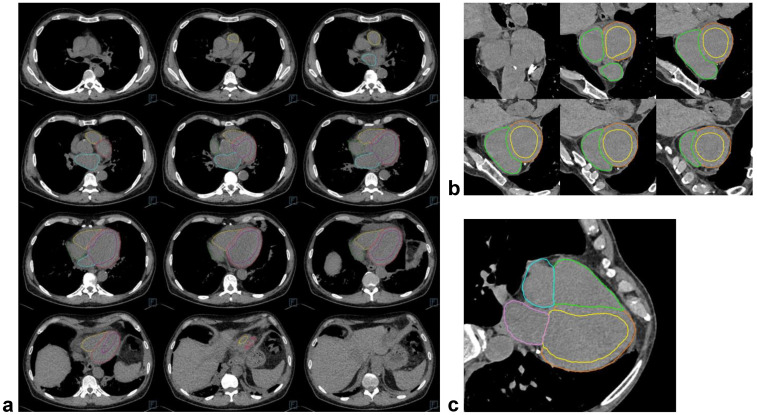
Examples of automatic cardiac chamber and myocardium segmentations on images from non-contrast chest CT scans. (a) Shows twelve axial slices from cranial to caudal for one example patient. (b,c) Show multiplanar reconstructions from another patient along the cardiac short-axis (ventricular base to apex, contours not displayed at the level of the annulus fibrosus due to the overlap of all four chambers) and long-axis in the 4-chamber view, respectively.
